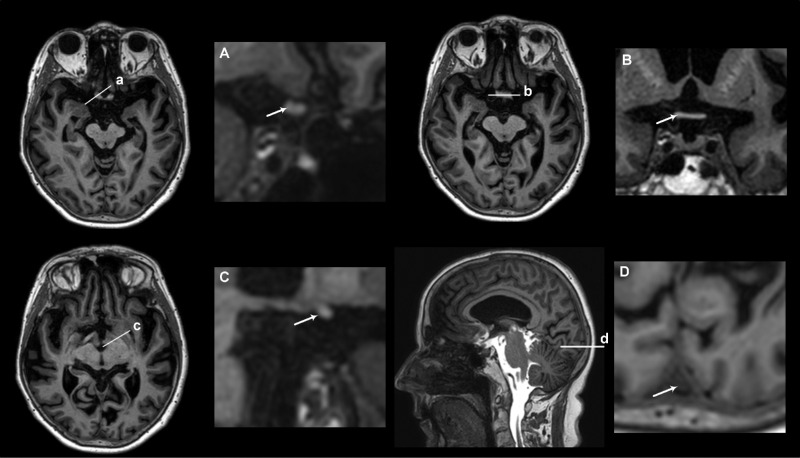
Anatomic location for placement of ROIs in the intracranial segment of the visual pathway in iNPH using T1-MRI. The figure demonstrates the approximate intracranial location along the visual pathway used for placement of the ROI. An anatomic overview is shown in the axial and sagittal planes where the letters a–d correspond with the magnified coronal slices (A–D). The following locations are shown and indicated by *arrows*: prechiasmatic part of optic nerve (*arrow*) (a/A), optic chiasma (b/B), optic tract (*arrow*) (c/C), and primary visual cortex and superior sagittal sinus (*arrow*) (d/D).
